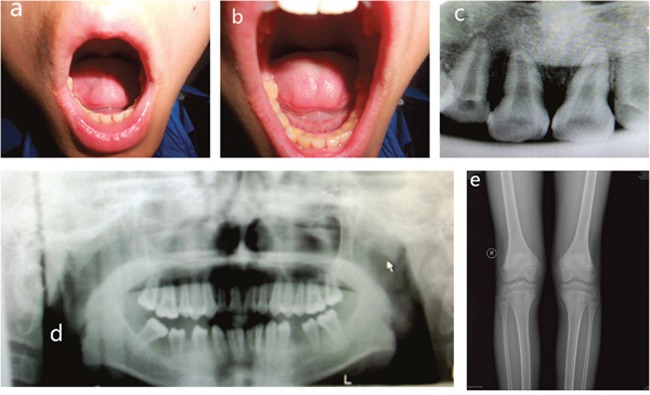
Clinical features of proband (A, B) Intra-oral photograph of proband showing that permanent teeth were erupted. (C, D) Panoramic radiographs showing normal permanent teeth. (E) Plain films of a knee joint presenting with metaphyseal osteoporosis changes.
